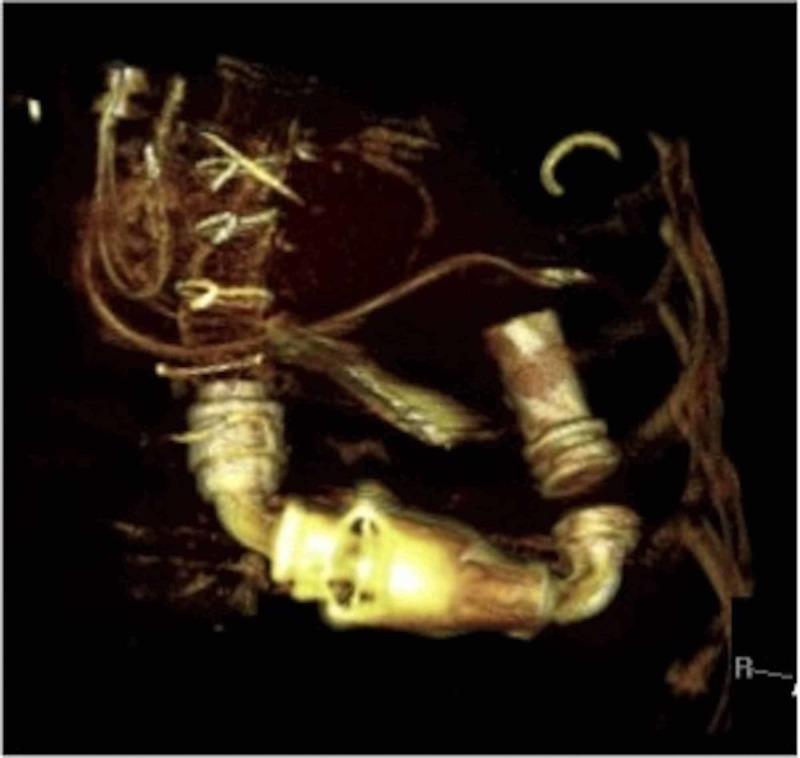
Volume rendered 3D computed tomography (CT) image showing parts of Heartmate II left ventricular assist device (LVAD) as described in Figure 1.
